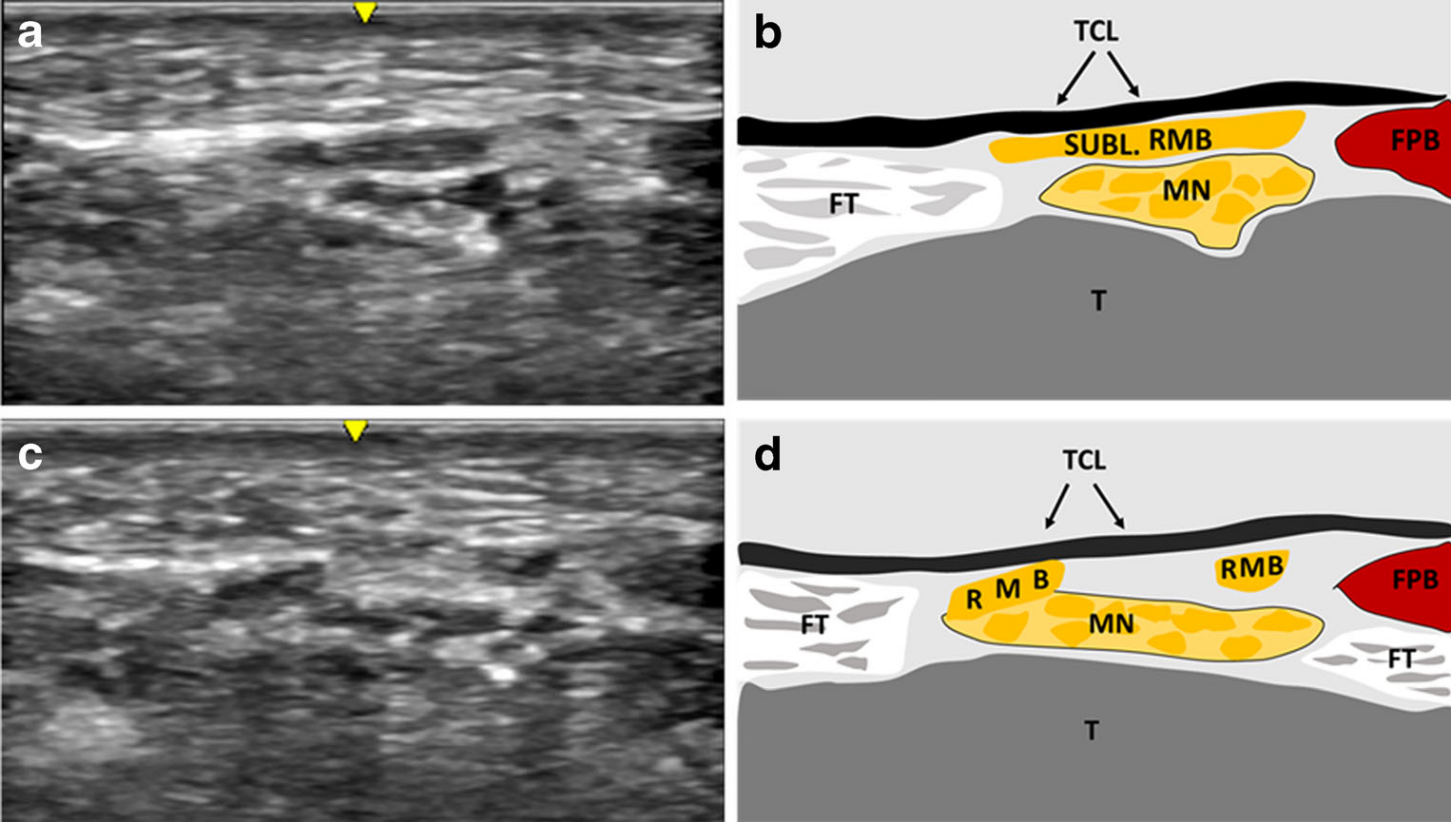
(a, b) Example of sonographic findings of the recurrent motor branch (RMB) originating from the ulnar aspect of the median nerve. (c, d) Example of sonographic findings in the same volunteer. The RMB crosses the anterior aspect of the median and courses beneath the transverse carpal ligament (TCL; subligamentous) toward the thenar musculature. *FPB* flexor pollicis brevis muscle, *FT* flexor tendon, *MN* median nerve, *SUBL* subligamentous
